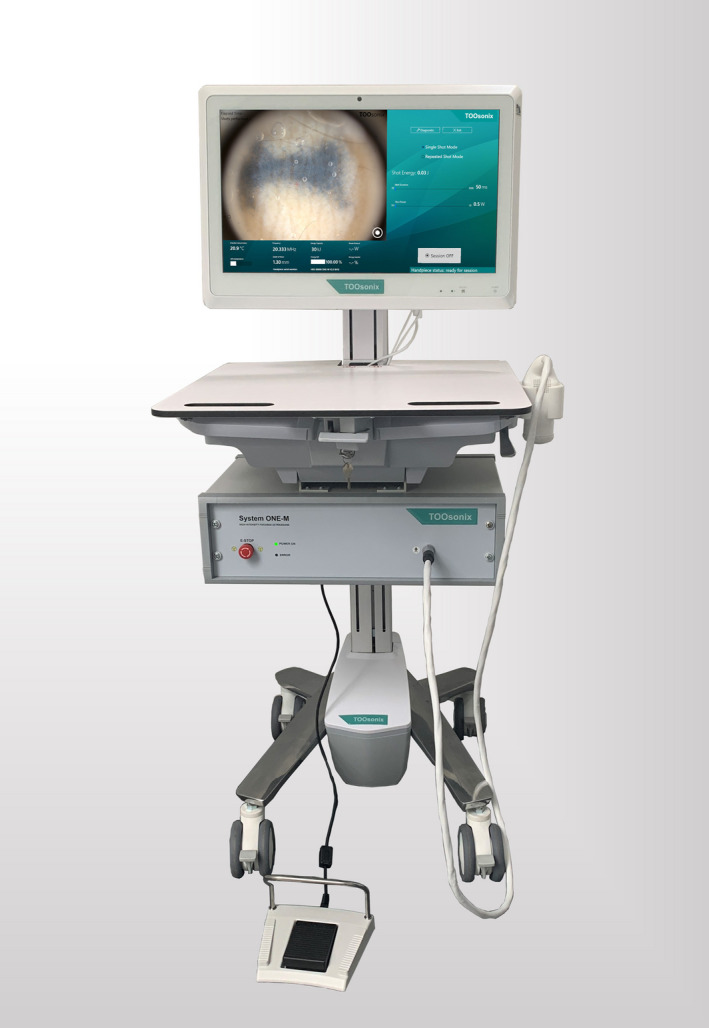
TOOsonix 20 MHz high‐intensity focused ultrasound (HIFU) System ONE used in the study for tattoo removal. A range of transducers and probes are available
